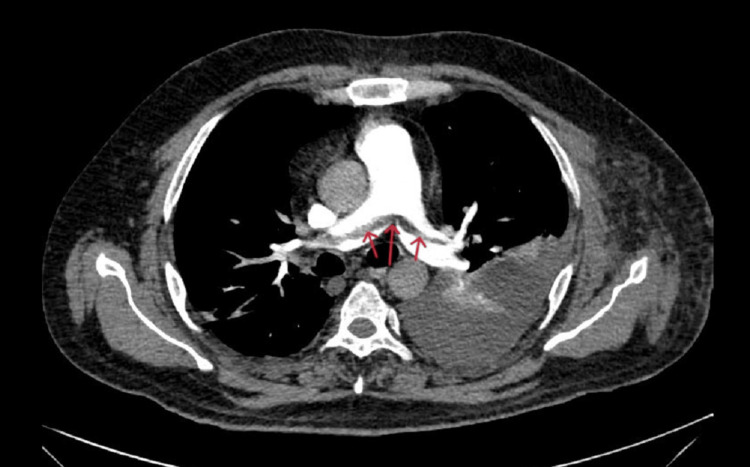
Axial section of CT pulmonary angiography showing hypodense filling defect (red arrows) at the bifurcation of the main pulmonary trunk extending to both right and left main pulmonary arteries.
